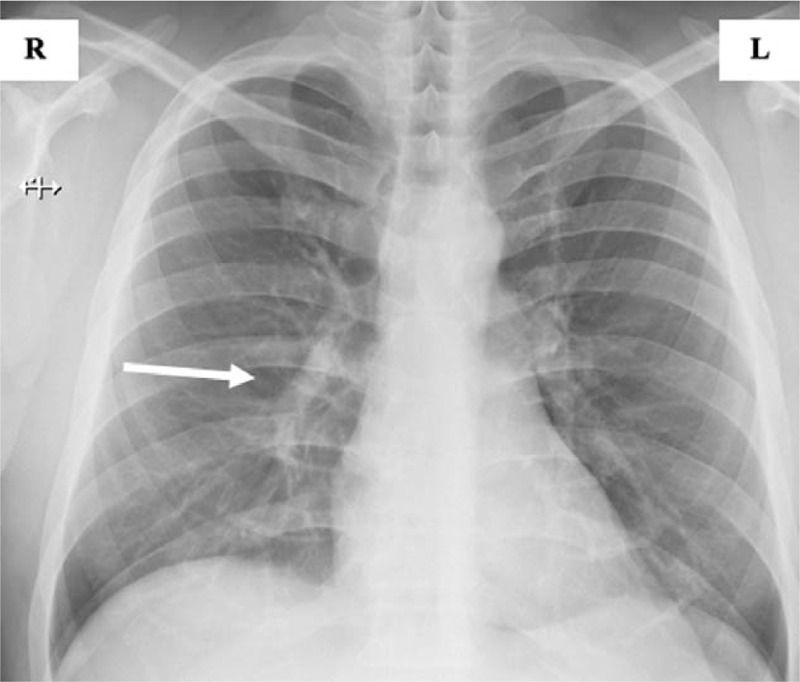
Chest X-ray before hospital discharge. Regression of inflammatory changes in the middle and lower lung field on the right (white arrow).
